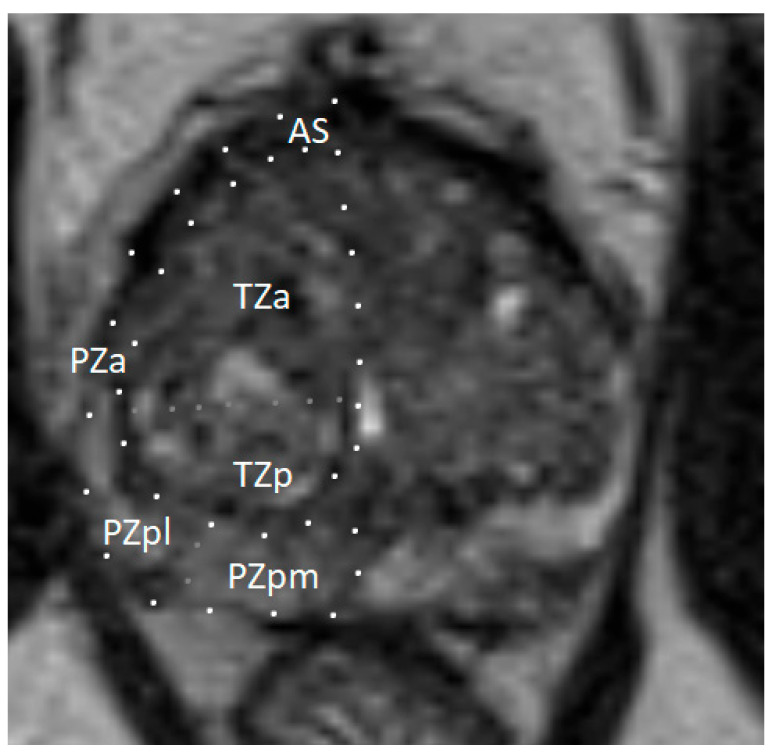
Zonal anatomy of the prostate gland on axial multiparametric magnetic resonance imaging (mpMRI). Adapted from the authors’ own materials, based on Yacoub and Oto [7]. a: anterior; AS: anterior [fibromuscular] stroma; p: posterior; pl: posterior lateral; pm: posterior medial; PZ: peripheral zone; TZ: transition zone.
